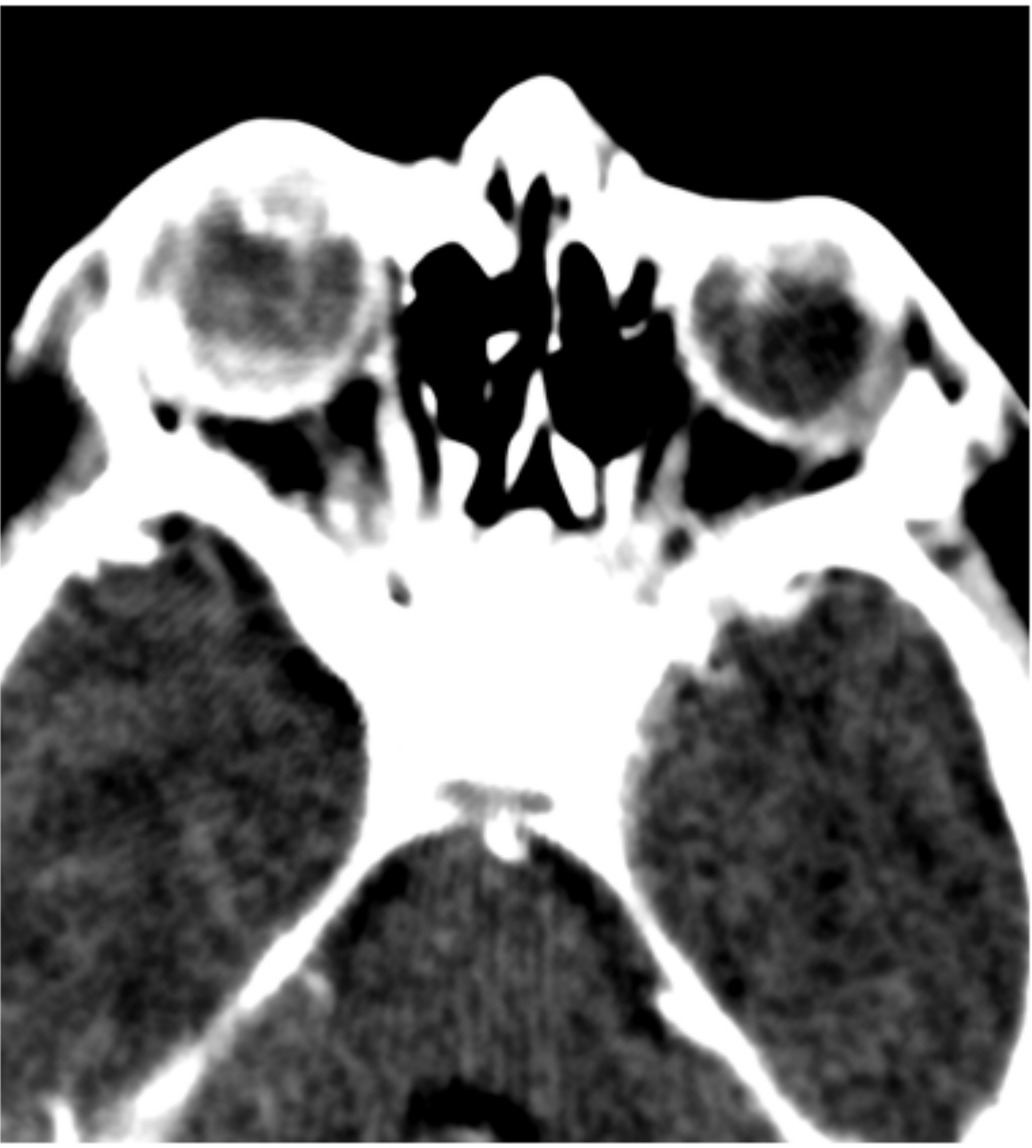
Post-contrast CT scan of both orbits showing a posterolental mass along with a thread-like extension in the posterior segment of the globes CT: computed tomography
